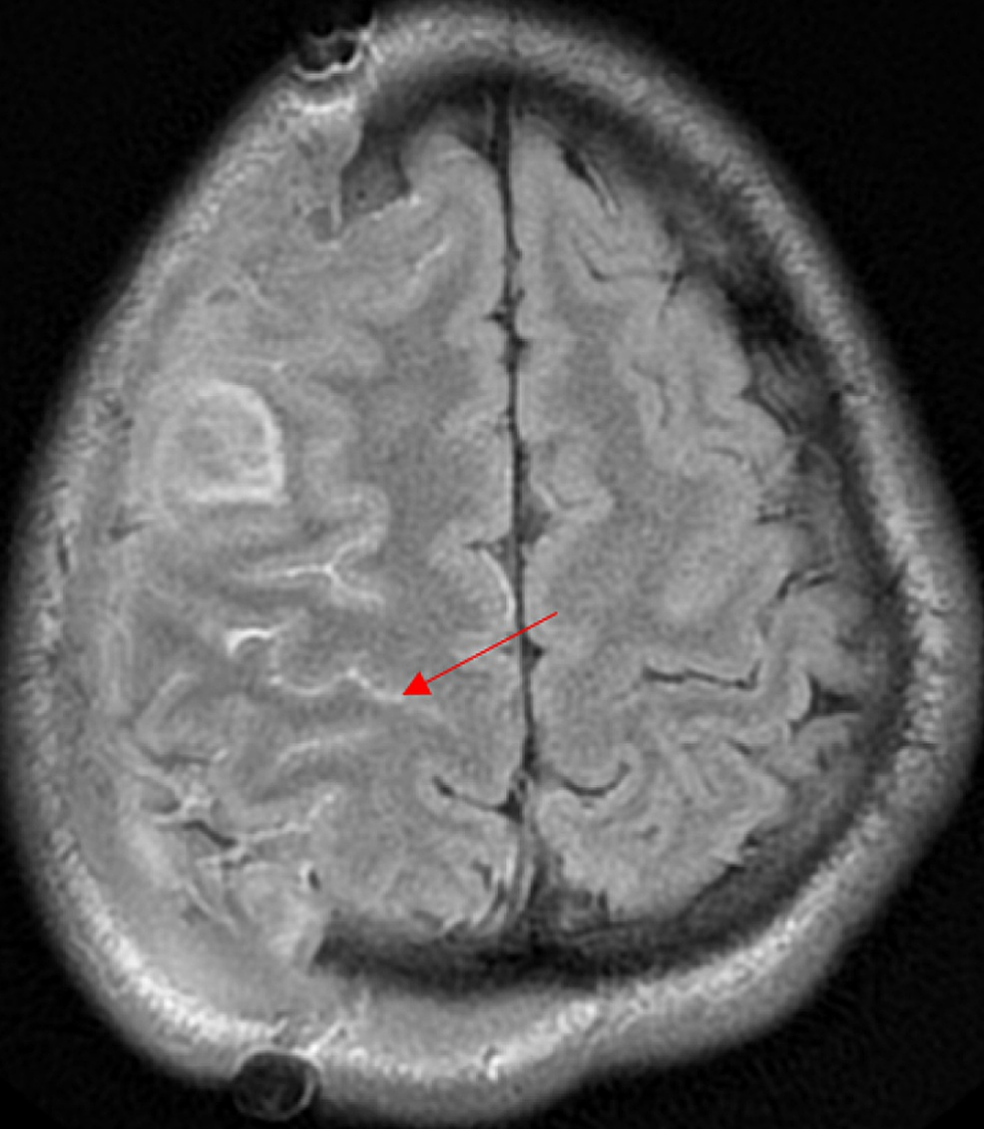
MRI brain with and without contrast post decompression, fluid-attenuated inversion recovery (FLAIR) sequence demonstrating subarachnoid hemorrhage in the right frontal and parietal lobe.
